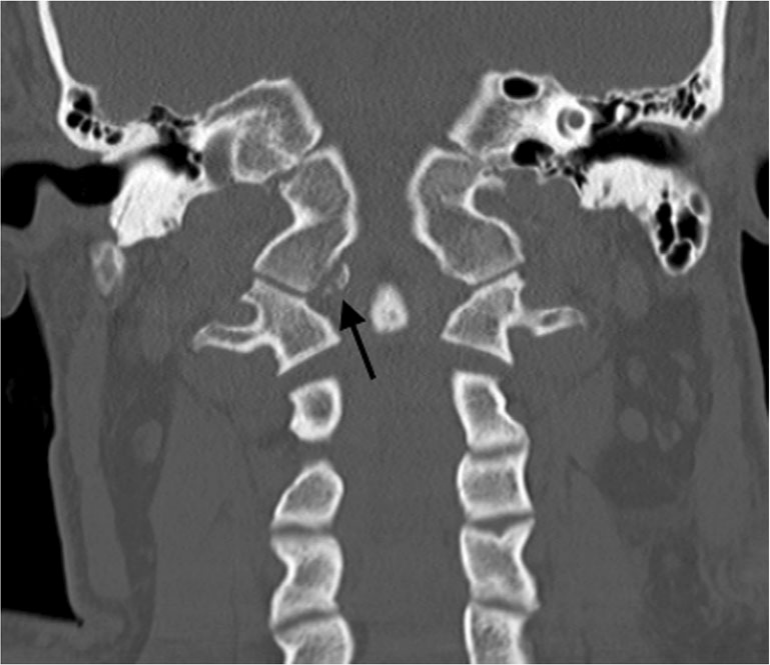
Type I occipital condyle fracture: coronal reformatted CT image through the craniocervical junction performed as part of a CT trauma assessment in a middle-aged male driver in a high-speed motor vehicle collision. A comminuted right occipital condyle fracture is present (*arrow*). The victim also sustained severe chest trauma and succumbed to his traumatic injuries the same day
